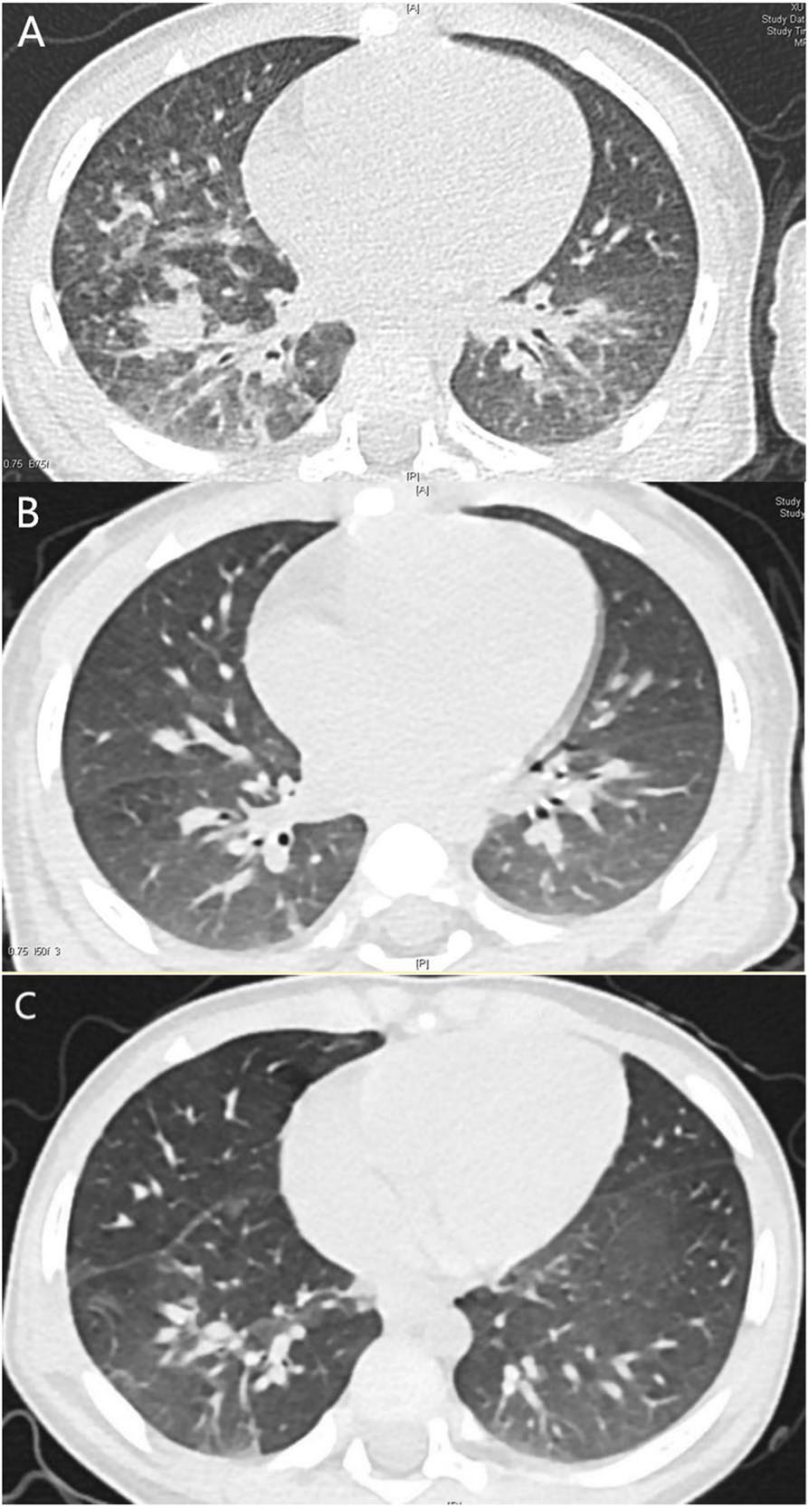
A Chest computed tomography (CT) scan. Image showing ground-glass shadows, diffuse grid-like shadows, multiple patchy shadows, thickened lung septum, and uneven brightness enhancement in both lungs on day 4. B Chest computed tomography (CT) scan. Image showing uneven brightness of the lungs along with multiple patchy shadows, blurred borders on day 10. C Chest computed tomography (CT) scan. Image showing reduced lung patchy shadows after treatment on day 50
